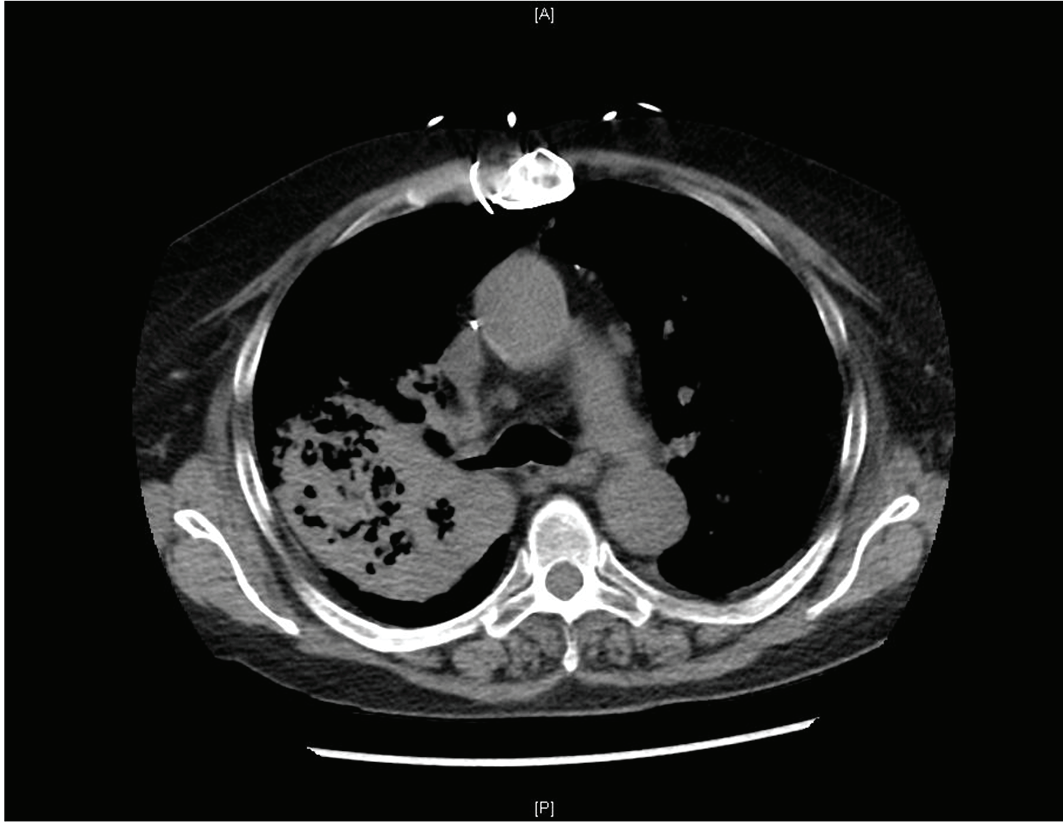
Initial chest CT of patient with bilateral nodular pulmonary infiltrates with predominance in the posterior right upper lobe.
